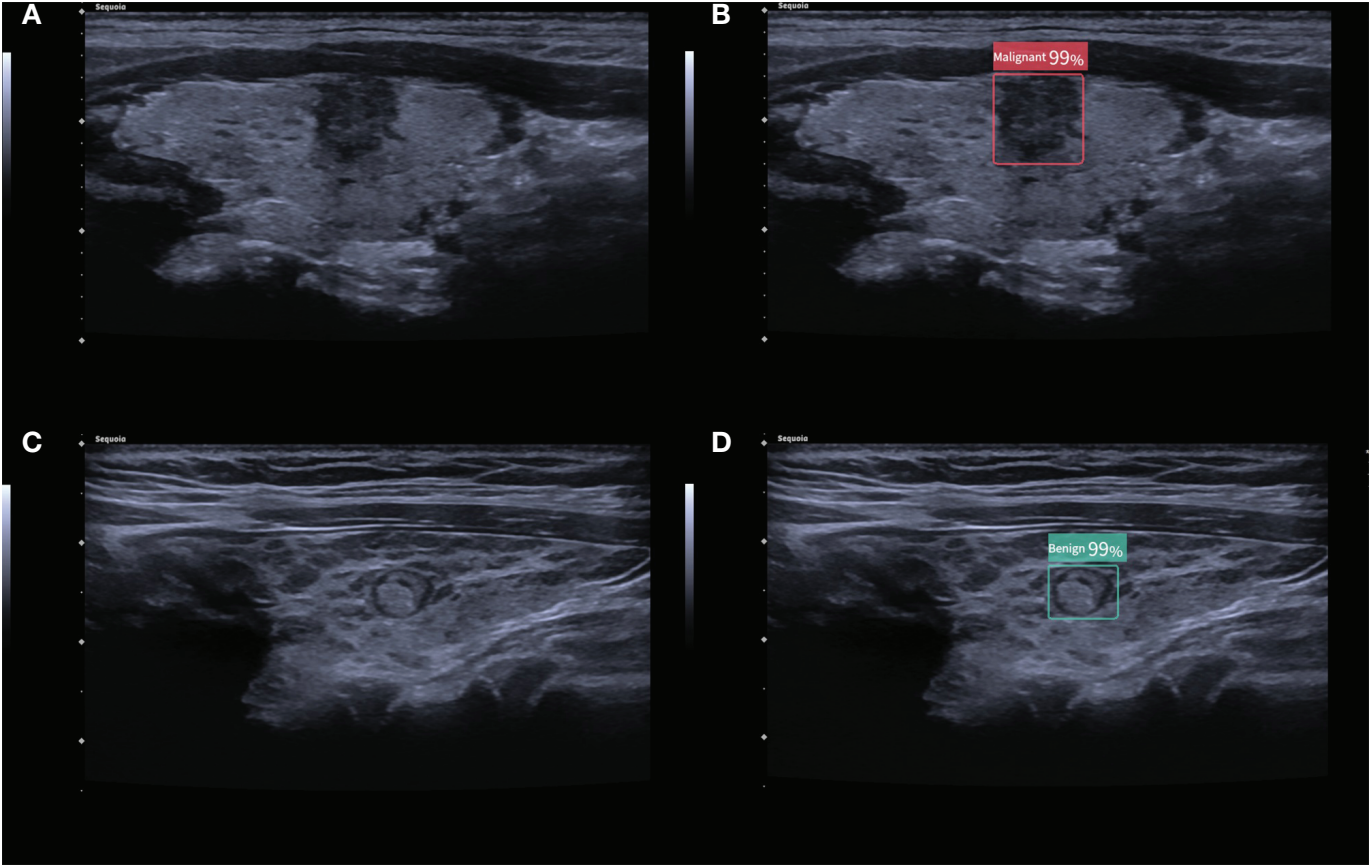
(A) 2D ultrasound image of a malignant thyroid nodule with HT; (B) the corresponding diagnostic results of the CAD system. (C) 2D ultrasound image of a benign thyroid nodule with HT; (D) the corresponding diagnostic results of the CAD system. The numbers in the figure are the benign and malignant rates produced by the CAD system. As the numbers from this CAD system are all greater than 95%, these numbers are not significant in our study.
